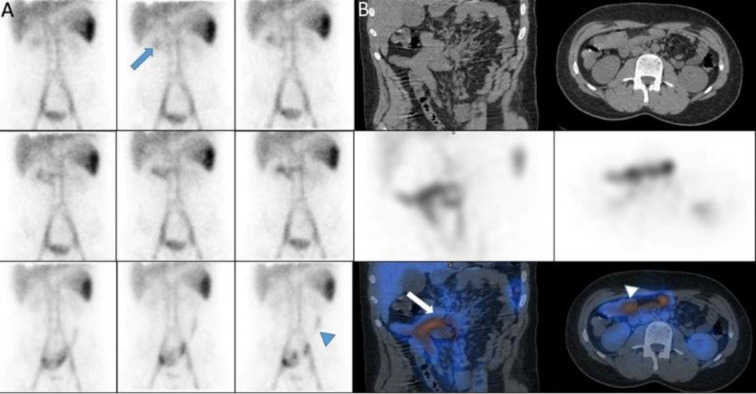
Dynamic (A) and SPECT/CT (B) images showed the source of bleeding is duodenum with rapid movement to jejunum (arrowhead)
